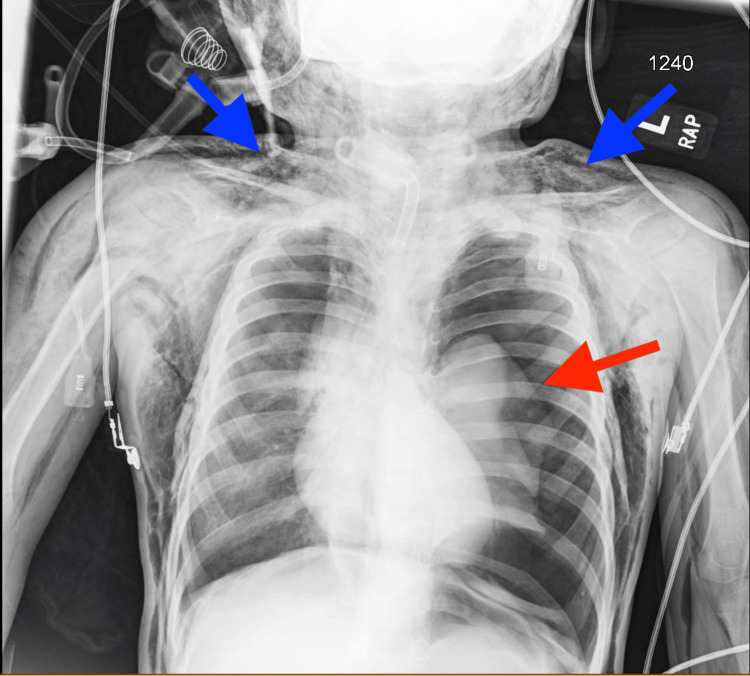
Initial chest radiograph findings Demonstrating a left-sided pneumothorax (red arrow) with extensive cervical and thoracic subcutaneous emphysema (blue arrows).
